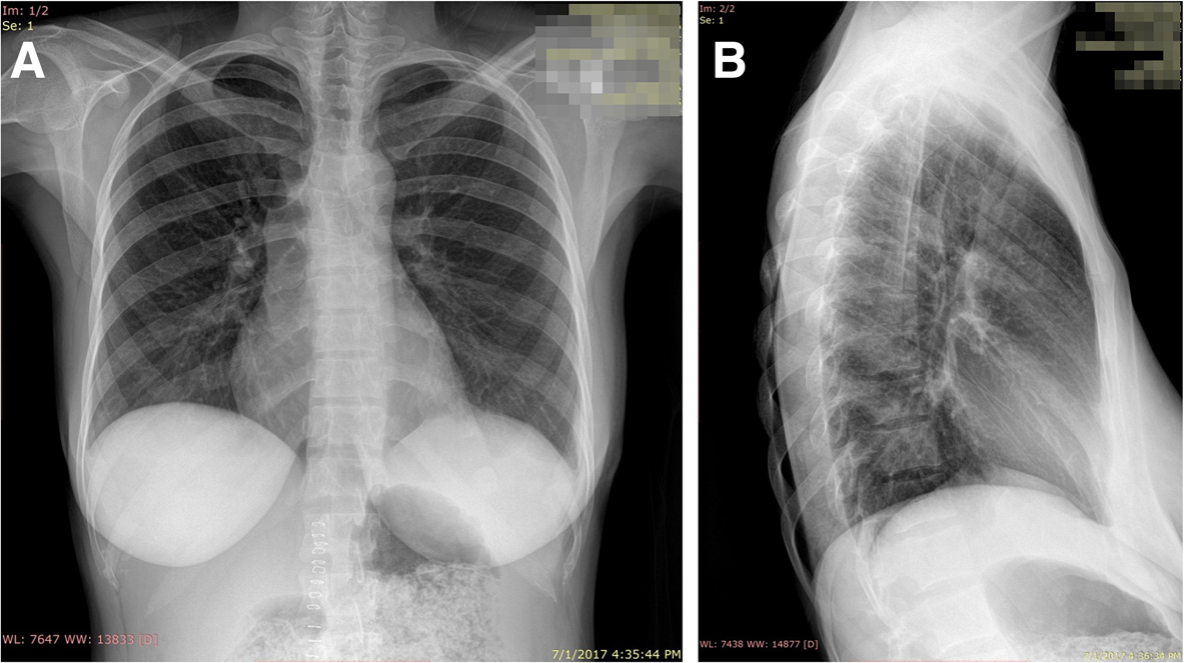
a and b One month follow up chest X-ray, completely normal
